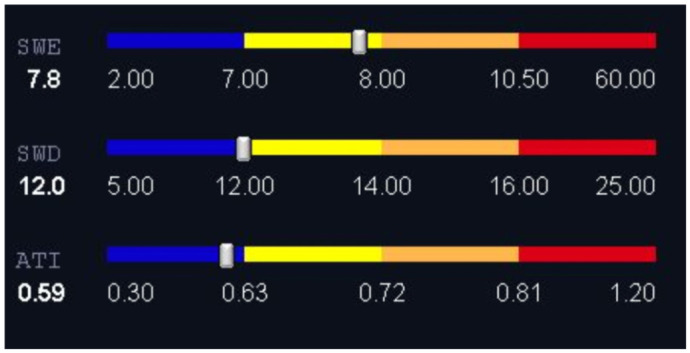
Case example of multiparametric ultrasound (mpUS) evaluation in patient with different results at initial and follow-up scan. MpUS in a female, 53 years of age (upon follow-up), with no history of previous liver disease. The initial mpUS scan revealed a slightly increased SWE value (median 7.8 kPa) and a borderline SWD value (12.0 (m/s)/kHz). Color coding of the horizontal bars defines severity grading of findings (blue—normal, yellow—light, orange—moderate, red—severe).
